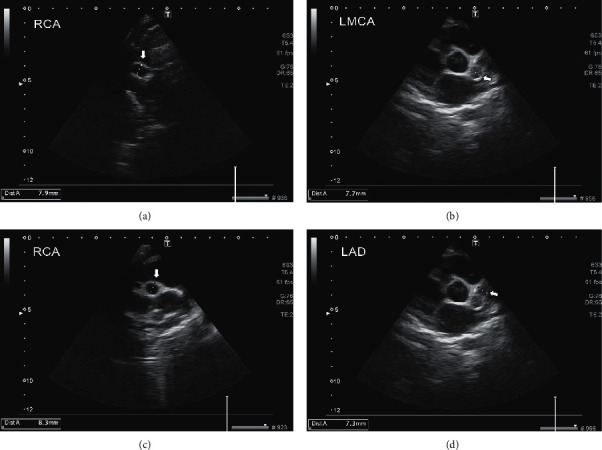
Echocardiography on the 20^th^ day of admission shows giant aneurysms in coronary arteries. (a) RCA, (b) LMCA, (c) RCA, and (d) LAD.
